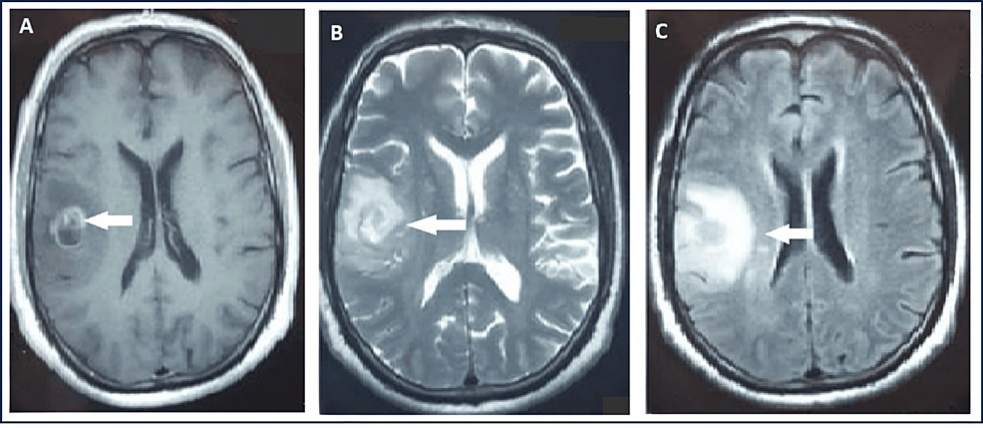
MRI aspect of a high-grade GBM in a contrast-enhanced T1-weighted axial image (A) with heterogeneous irregular peripheral enhancement associated with the right parietal lobe mass and central non-enhancing area, consistent with necrosis and associated mass effect on the right lateral ventricle, T2 (B) and flair sequence (C) showing a heterogeneous mass centered in the right parietal lobe with surrounding infiltrating signal abnormality Arrows highlight the lesion on the different sequences.
